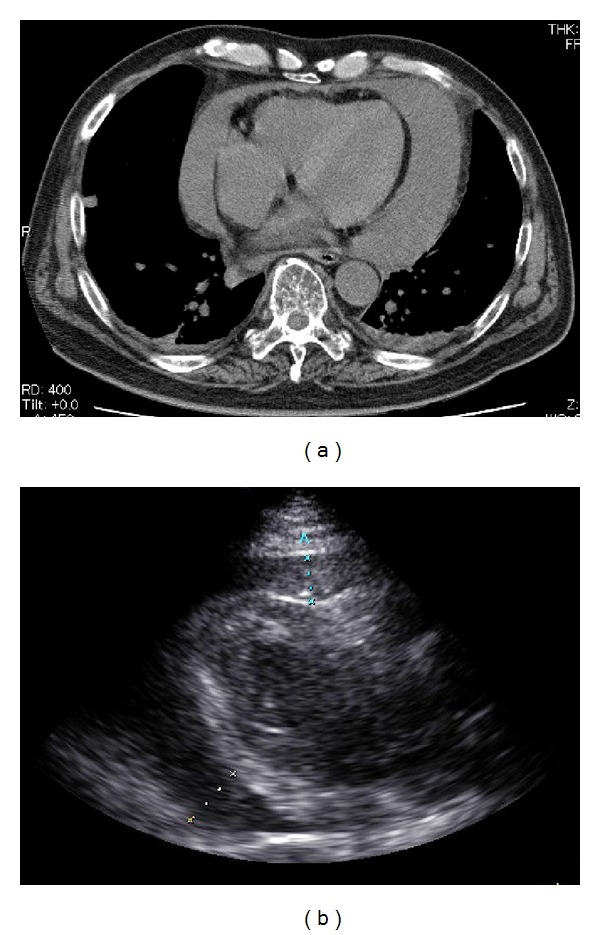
Chest CT scan showing large pericardial effusion (a) and echocardiogram showing circumferential pericardial effusion (b).
